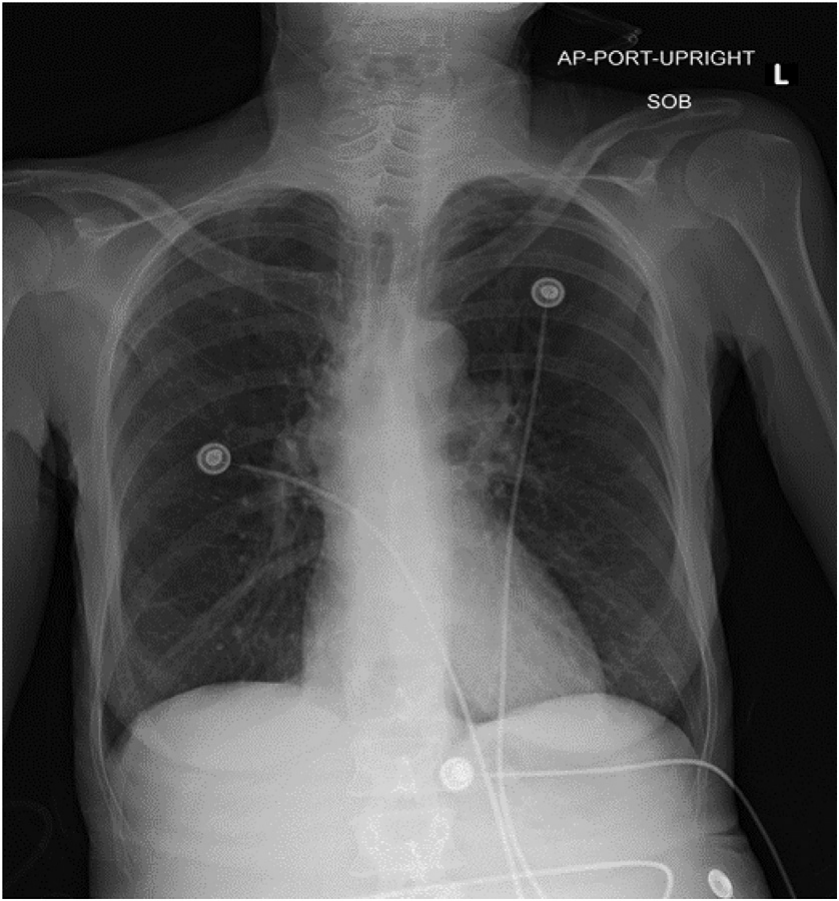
Chest radiograph of a 54-year-old woman with chest pain.
